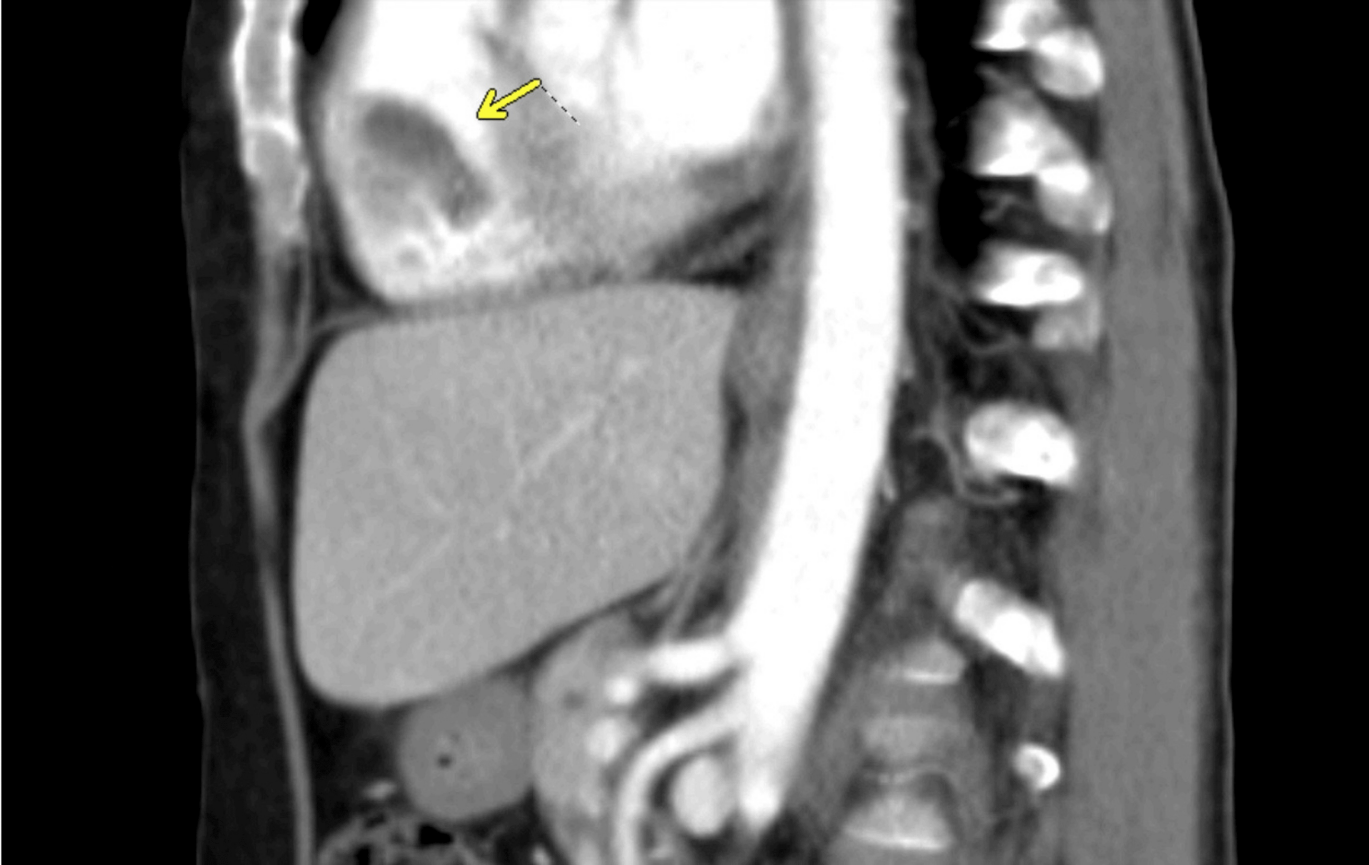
Repeat sagittal post-contrast CT of the chest showing persistent right ventricular thrombus (arrow), unchanged in size and morphology.
